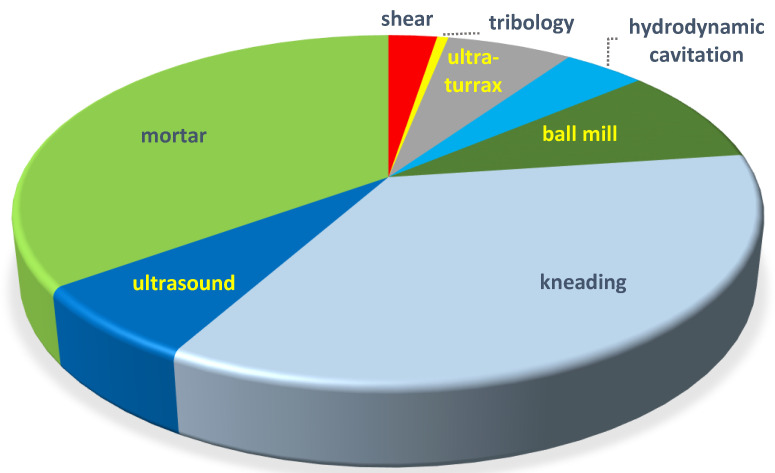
Distribution of various complexations in the technical papers till 28 July 2021. Due to the overlaps of some topics, such as grinding and kneading, hydrodynamic cavitation/ultra-turrax, and ultrasonic mixing, the percentages carry little information.
